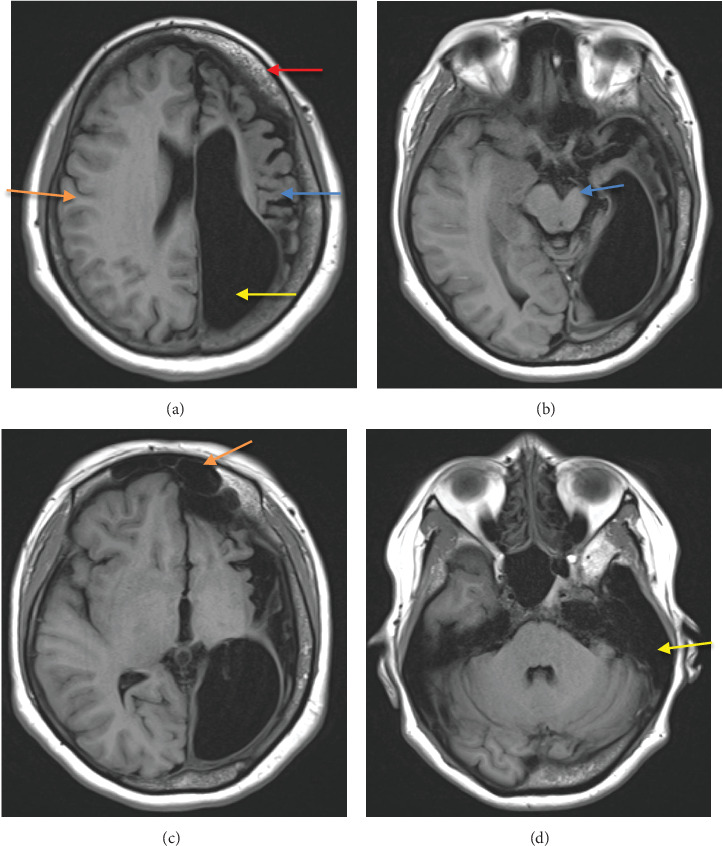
The T1-weighted axial image at the level of the central semiovale shows diffuse left cerebral atrophy (blue arrow [a]) with hypertrophy of the right cerebral hemisphere (orange arrow [a]), ex vacuo dilatation of the left lateral ventricle (yellow arrow [a]), and diffuse left calvarial thickening (red arrow [a]). The T1-weighted axial image at the level of the midbrain shows atrophy of the left cerebral peduncle consistent with Wallerian degeneration (blue arrow [b]). The T1-weighted axial image at the level of the frontal sinus shows hypertrophy of the left frontal sinus (orange arrow [c]). The T1-weighted axial image at the level of the bilateral petrous apex shows hypertrophy of the left mastoid sinus (yellow arrow in d).
